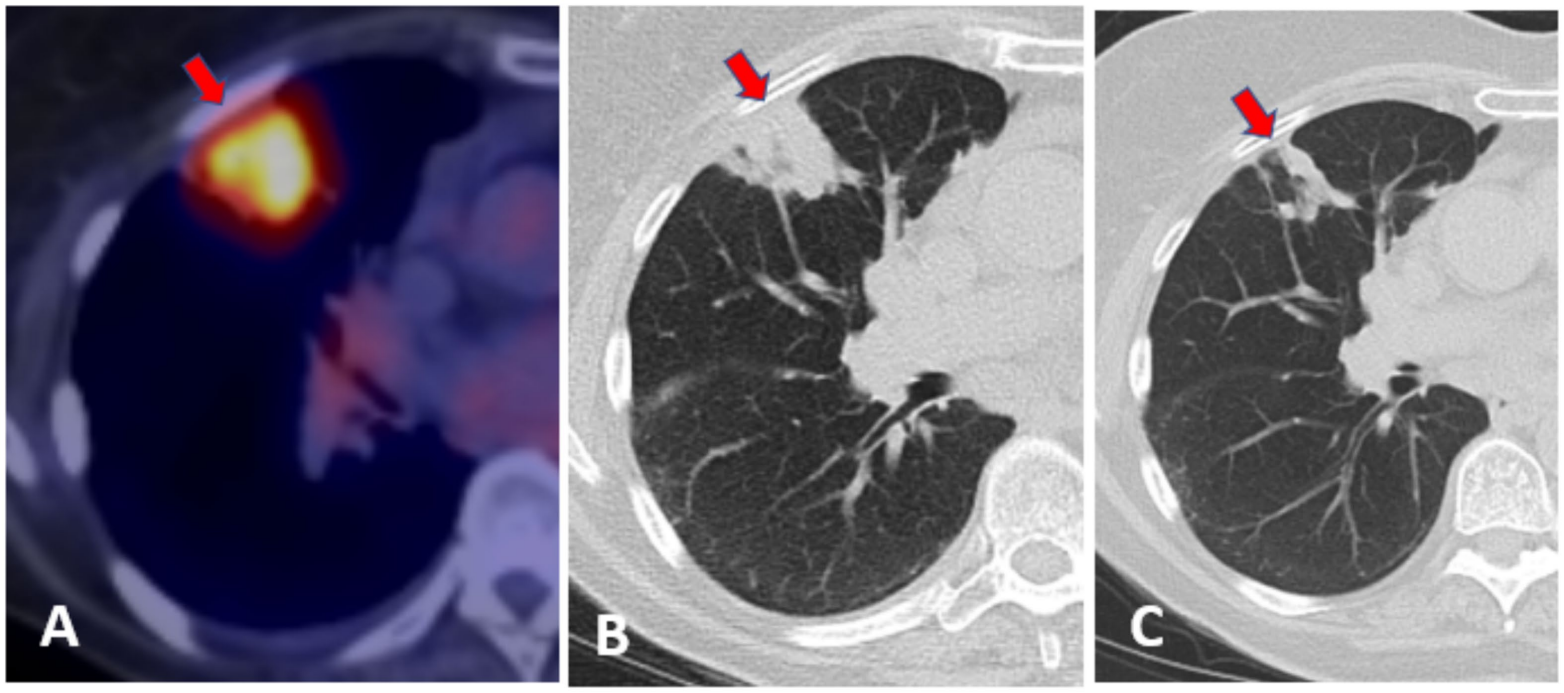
(A) Initial FDG-PET scan 27 April 2021. (B) Pre-treatment CT scan 9 September 2021. (C) Post 6-month treatment CT scan 3 March 2022.
